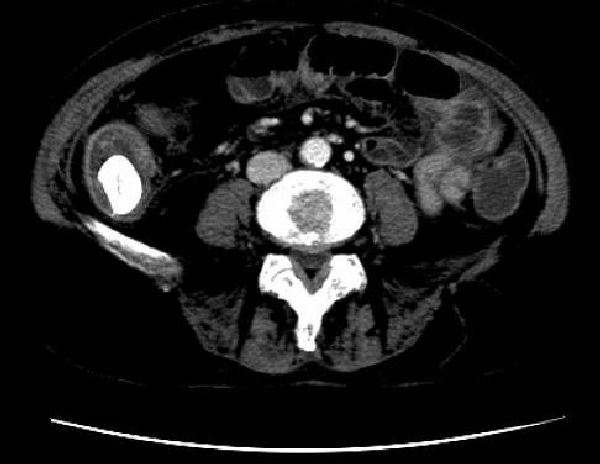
The CT scan of the abdomen showing gall stone in the small bowel lumen with no signs of bowel obstruction, The bowel wall showing proximal edema to the gall stone.
